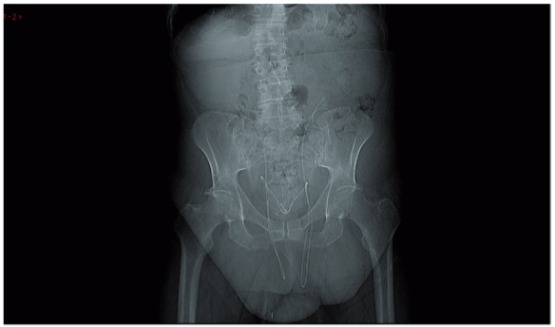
CT-KUB scout film. The stents outline the unusual anatomical passage of both ureters. The upper coil of the stents shows the renal pelvises have been pulled down to L4/L5vertebral level.
